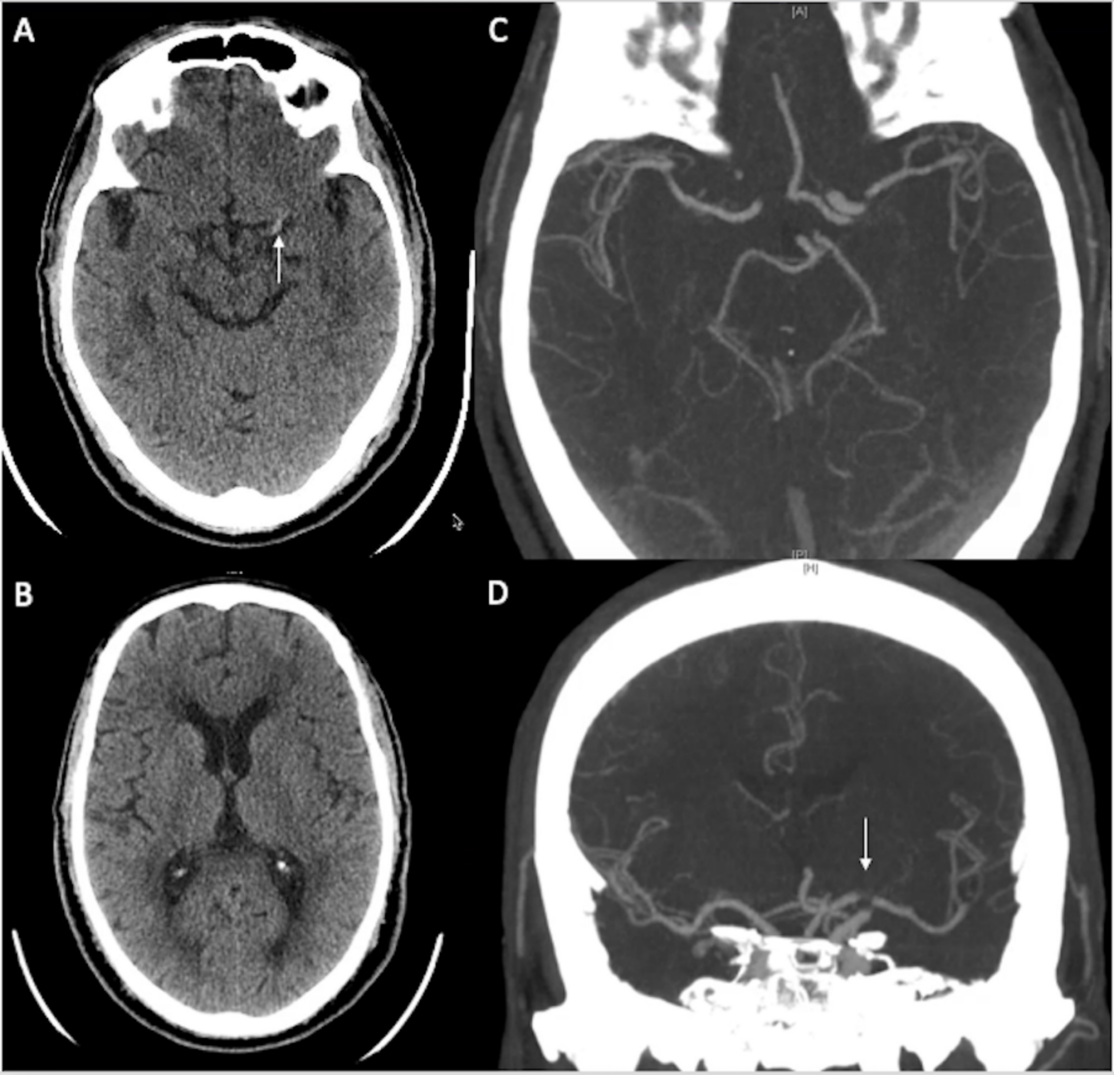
Case 2: neurological imaging. (A) CT of the head shows a left MCA hyperdense sign (white arrow). B) CT of the head shows no wide extension of left MCA territory infarct. (C, D) CTA of the head shows the location of left MCA occlusion. MCA, middle cerebral artery; CTA, CT angiography
